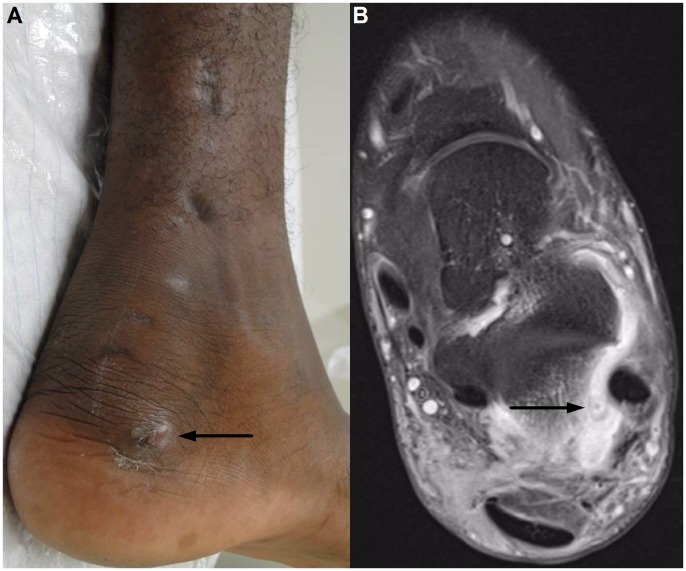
Case 2. (A) Clinical presentation after drainage of the subcutaneous abscess (arrow). (B) T2 fat-saturated MRI reveals diffuse inflammation of the soft tissue and a pathognomonic central hypointense dot (arrow) surrounded by hyperintensities reflecting the grain.
